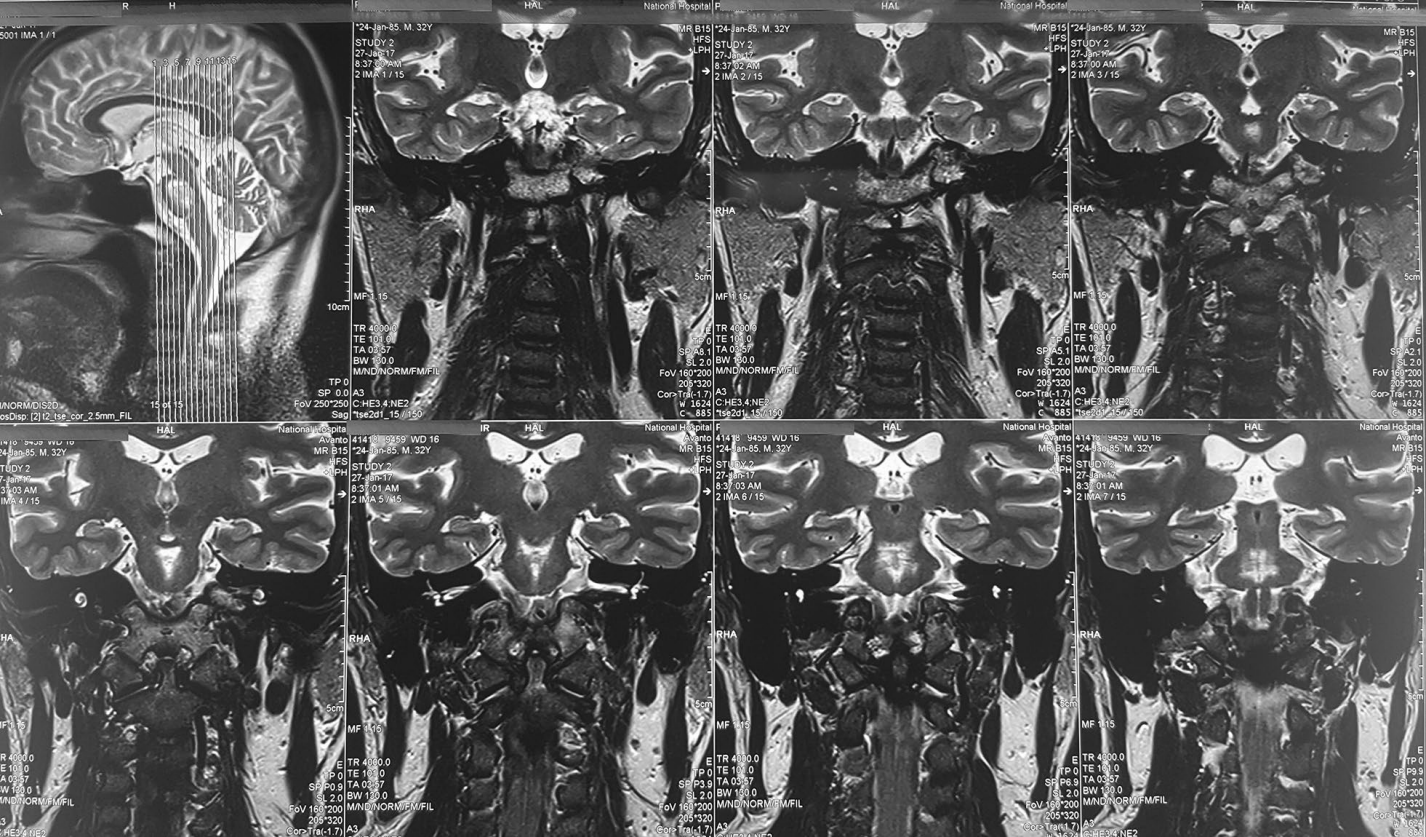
MRI of the brain stem, done 1 month after the first MRI coronal views, showing high signal intensities localized to the pons and not extending into the medulla
